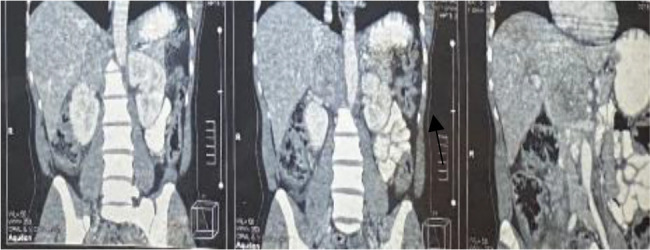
Abdominal CT scan; coronal section
